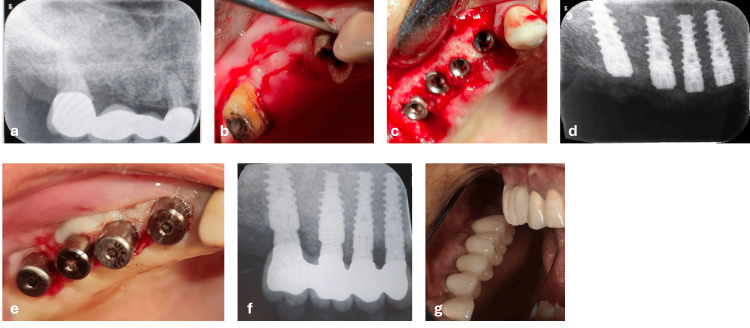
Second surgical stage and prosthetic rehabilitation of case 1. (a) Periapical radiograph obtained four months after sinus augmentation. (b) Extraction of hopeless teeth in the posterior maxilla. (c) Intraoperative view showing placement of four dental implants. (d) Postoperative radiograph confirming the placement of implants. (e) Healing caps placement. (f, g) Demonstrate the final prosthetic rehabilitation with a fixed prosthesis supported by four implants, restoring the posterior maxillary dentition.
